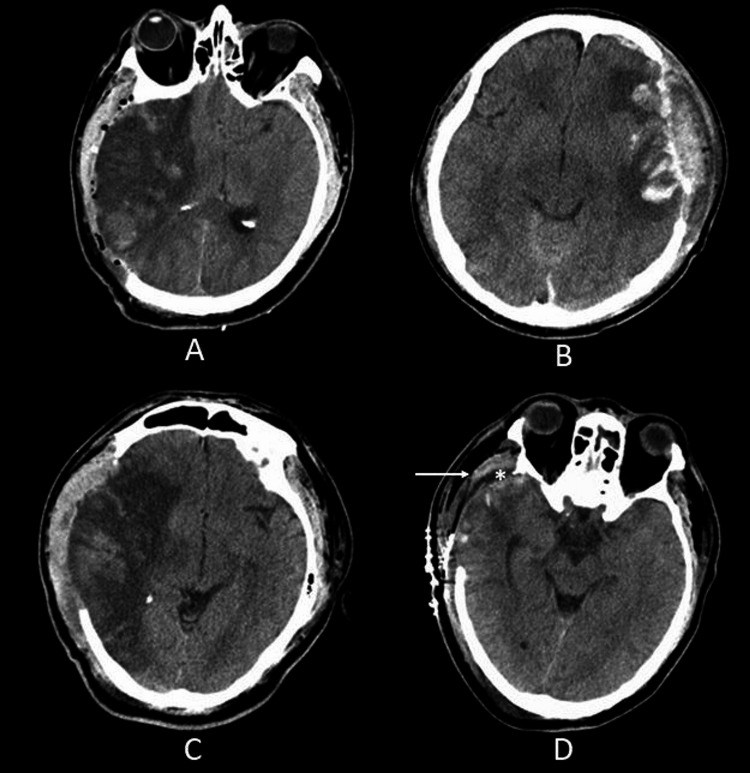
Representative axial computed tomography (CT) slices showing: swollen right temporalis muscle (A); left temporalis hematoma with indentation of the dura, brain compression, and midline shift (B); swollen right temporalis muscle and subgaleal hematoma (C); preserved right temporalis muscle (arrow) with additional space (asterisk) over the temporal lobe occupied by CSF (D). Image courtesy Dr. Salazar Jones.
